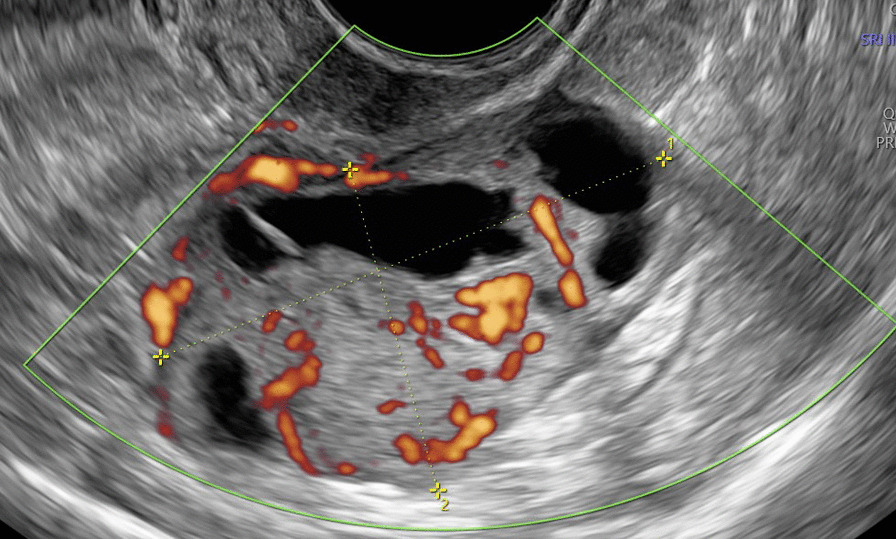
Power Doppler transvaginal ultrasound image of a solid inhomogeneous mass (solid component > 80%) with multiple anechoic cysts, irregular external contour and with a remarkable vascularization at Power Doppler (Color Score 4)
